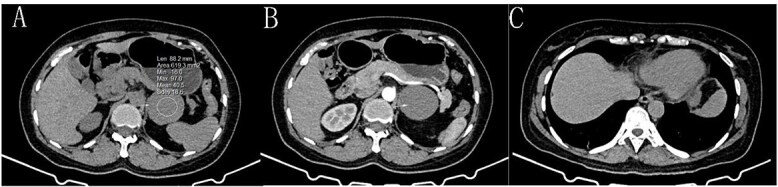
A: Gastric bronchogenic cyst on a plain CT scan (→), with a CT value of approximately 40.5 HU. B: The CT-enhanced arterial phase shows no enhancement of the cyst (→). C: A postoperative plain CT scan shows that the cyst has been successfully removed.
